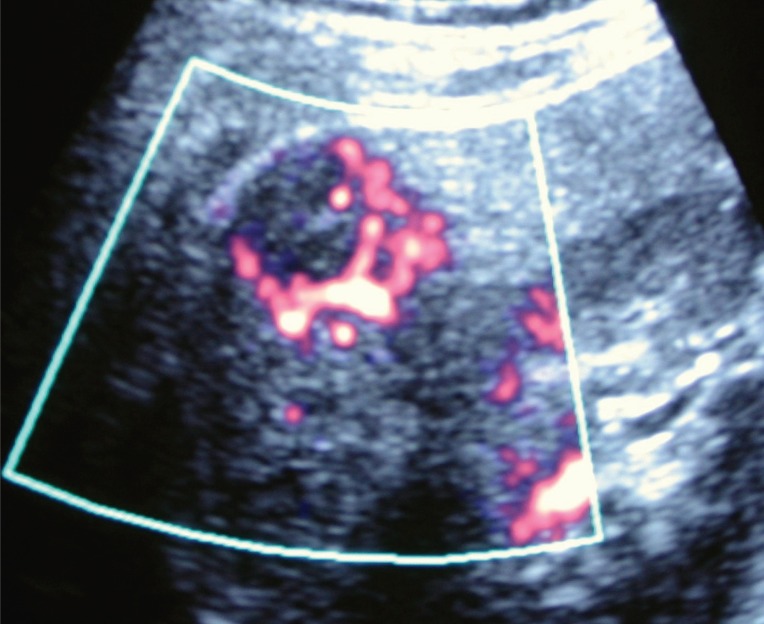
Power Doppler showing flow signals in and around the nodule of the segment 6 of the liver.
